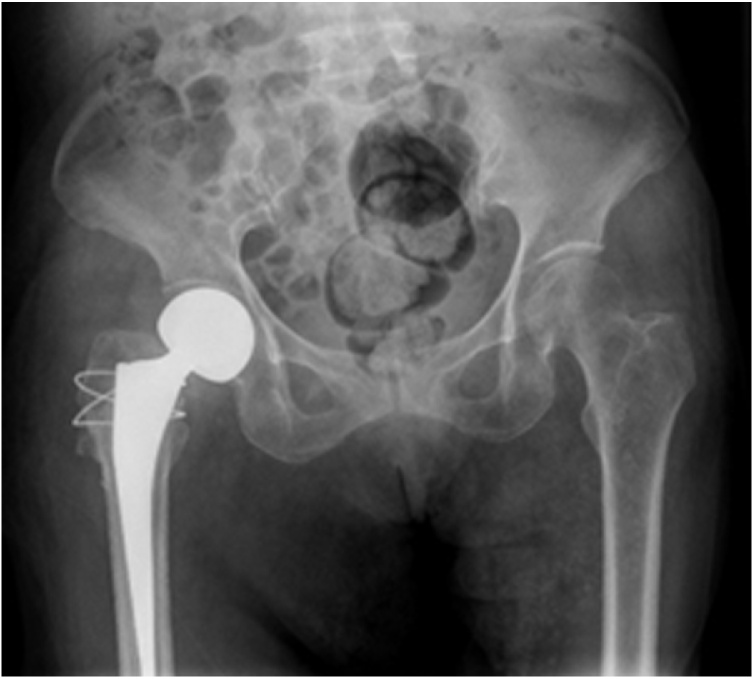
Postoperative radiograph demonstrating the revision hip hemiarthroplasty.
